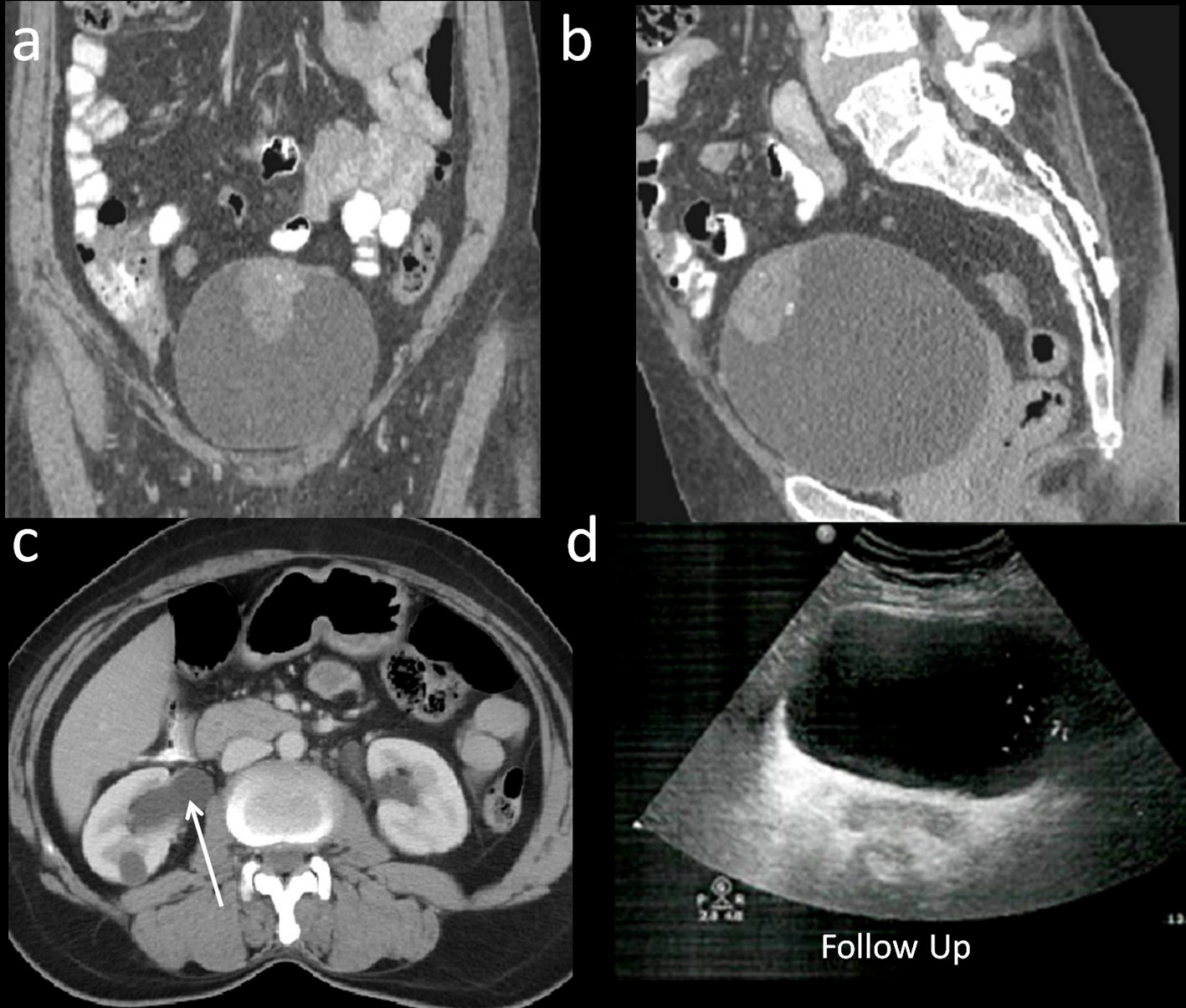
46-year-old-female with haematuria. Coronal and Sagittal reconstructions of urinary bladder in venous phase (a, b) shows filling defect in urinary bladder containing calcific foci. There is minimal thickening of bladder wall on left side. Axial image at the level of kidneys show mild dilatation of right renal pelvis (arrow). 6 month follow-up sonogram (d) of urinary bladder shows normal lumen and contours of urinary bladder.
